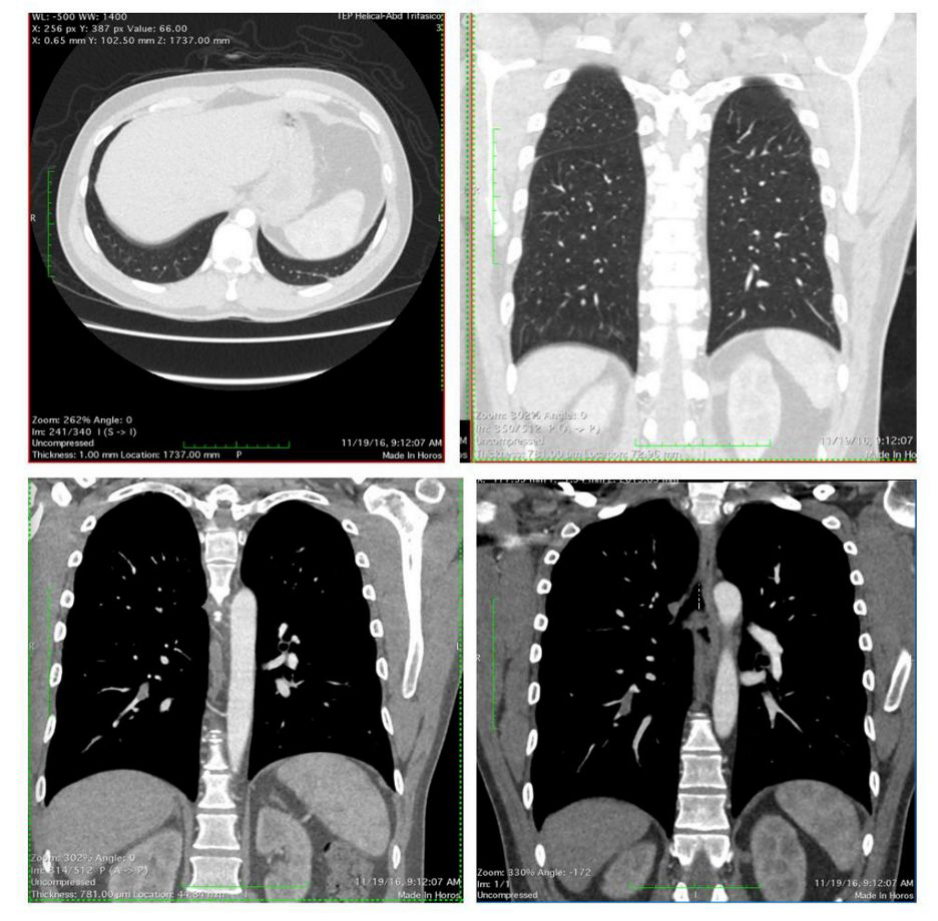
CTA diagnosing segmental and sub-segmental thrombus in the right lung.
